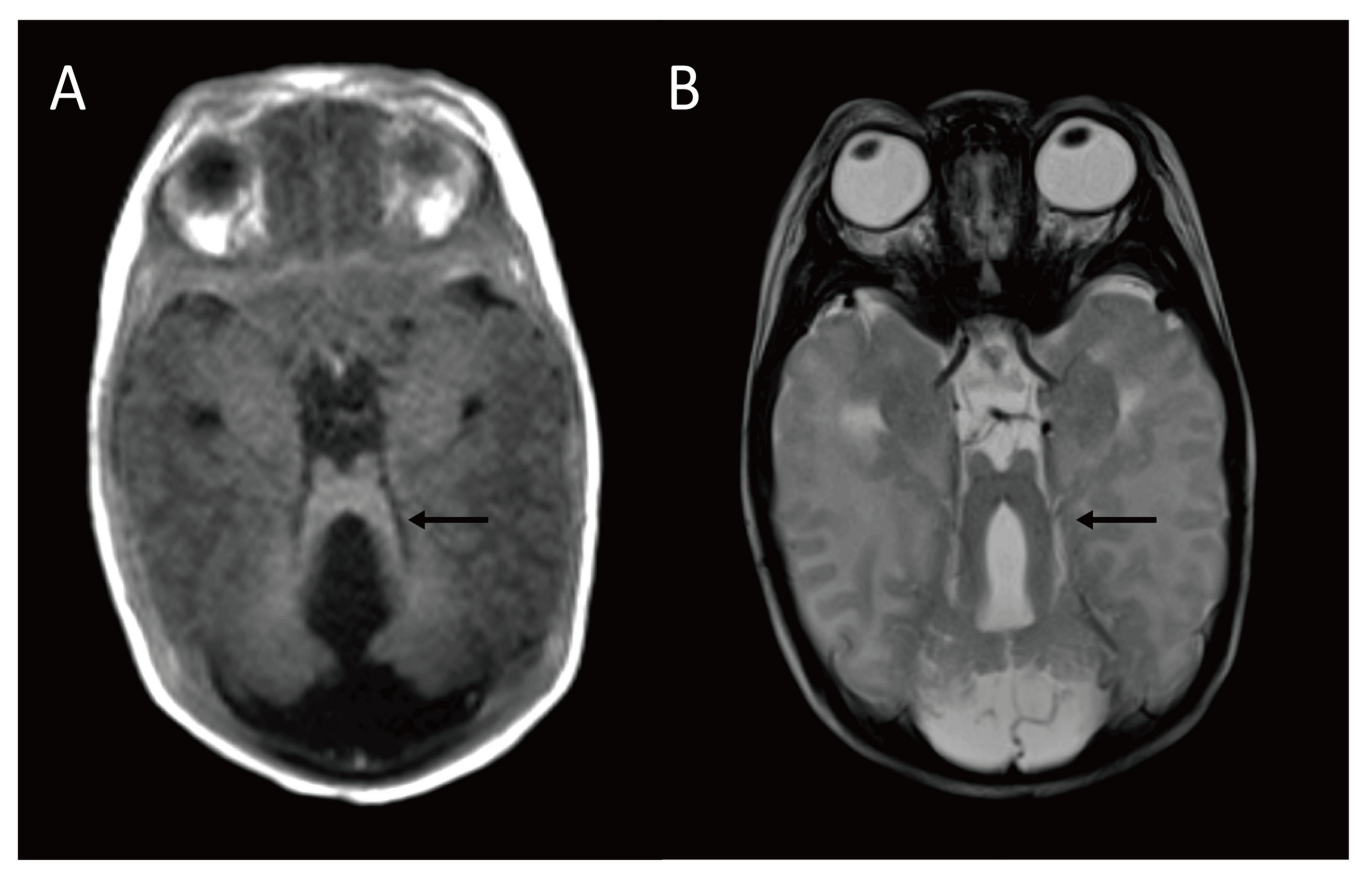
Magnetic resonance imaging of the brain revealed the molar tooth sign. (A) Axial T1-weighted image; (B) axial T2-weighted image.
